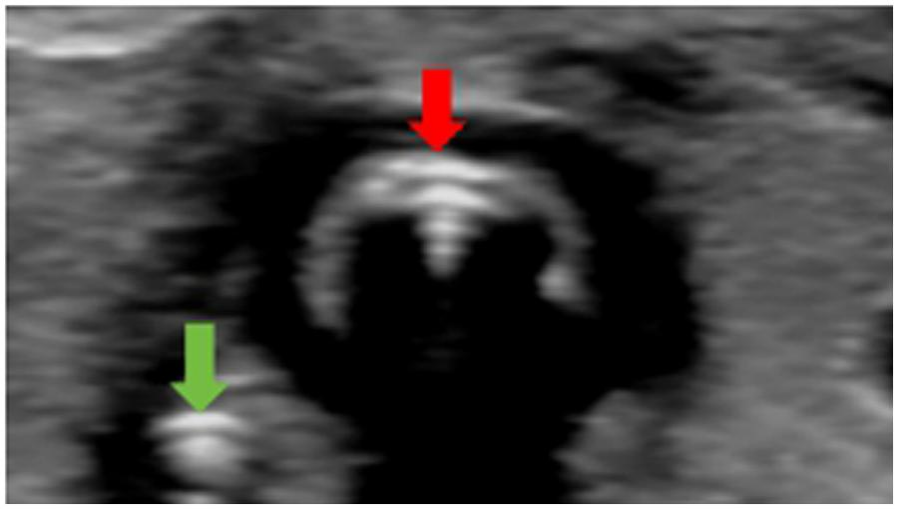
Using a linear probe for transverse scanning of the anterior neck, a double-track sign (indicated by the red arrow in the image) is visible in the transverse section of the trachea. The thyroid glands are located on both sides of the trachea. The green arrow in the lower left corner of the image indicates the position of the esophagus, which is adjacent to the trachea. Subsequently, a gastric tube was inserted, and the double-track sign was also observed under ultrasound.
